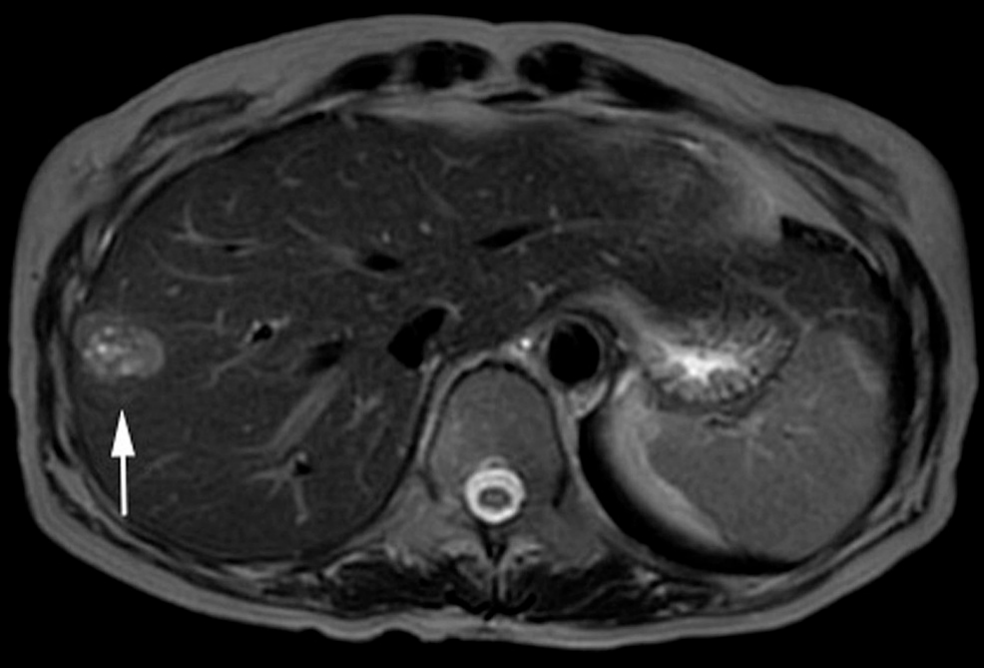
Abdominal MRI. Gadolinium ethoxybenzyl diethylenetriamine pentaacetic acid-enhanced MRI showed the 28-mm-sized hepatic tumor (arrow) on T2-weighted imaging. MRI: magnetic resonance imaging
